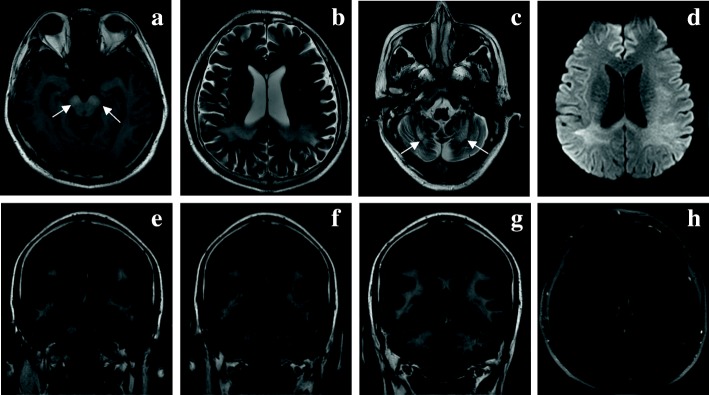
MRI of the brain images of patient 6 with AMN, shown rapidly deteriorated symptoms with cognitive decline. a The lesion showed increased signal intensity with bilateral corticospinal tract (arrows) on T1-weighted images. b-c The extensive lesions involved the parieto-occipital white matter, splenium of the corpus callosum and bilateral dentate nuclei of cerebellar (arrows) on T2-weighted images. d The lesion showed slightly increased signal intensity with the parieto-occipital white matter and splenium of the corpus callosum on Diffusion-weighted images. e-g The extensive lesions involved the bilateral temporo-parietal-occipital white matter, splenium of the corpus callosum, bilateral corticospinal tract and bilateral dentate nuclei of cerebellar on T2-flair images. h Axial T1‑weighted images after gadolinium administration
